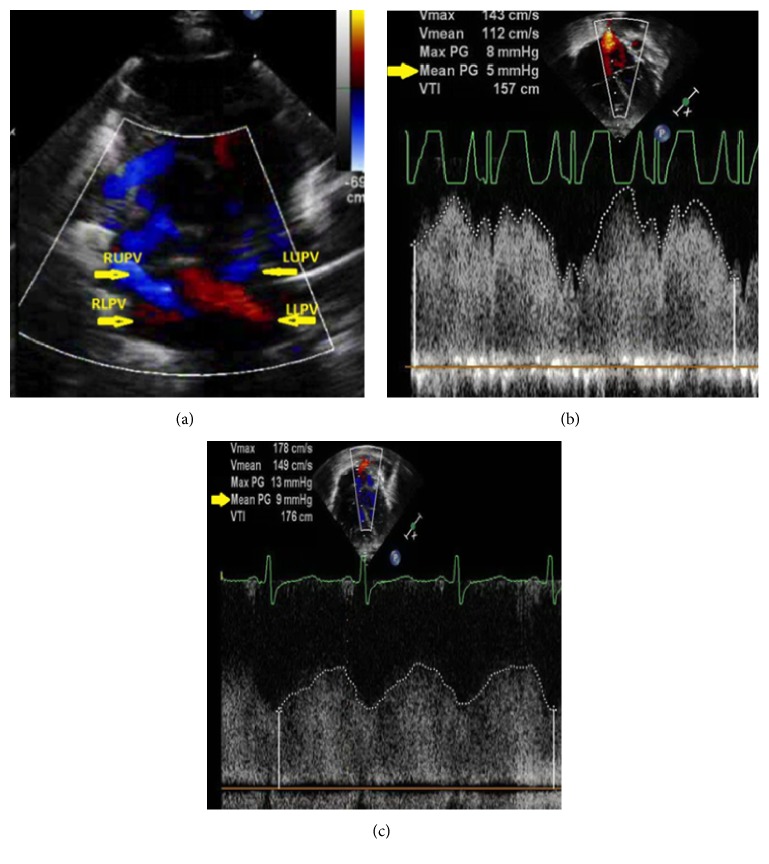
Transthoracic echocardiogram images demonstrating progressive pulmonary vein stenosis. (a) Color flow Doppler of pulmonary veins at initial diagnosis, with (b) measured mean pulmonary gradient (mean PG) of 5 mmHg and (c) increased mean pulmonary pressure gradient to 9 mmHg by 6 weeks after diagnosis.
